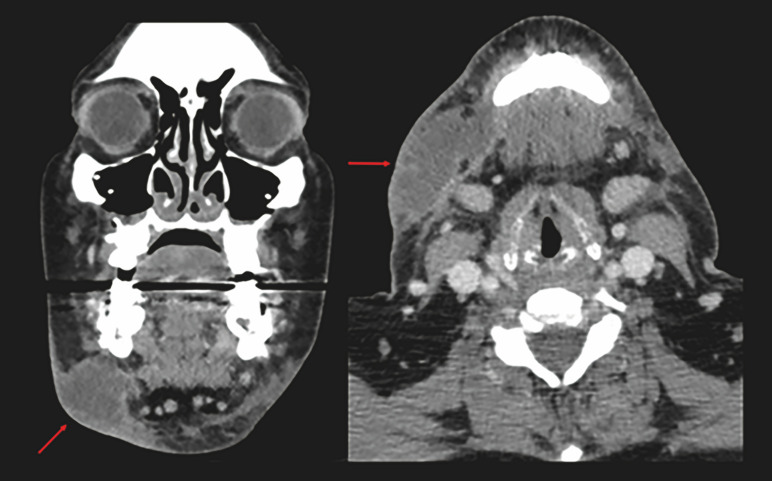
CT scan of the neck with coronal and axial contrast. Right perimandibular collectionis visualized in relation to the area with infectious process.
